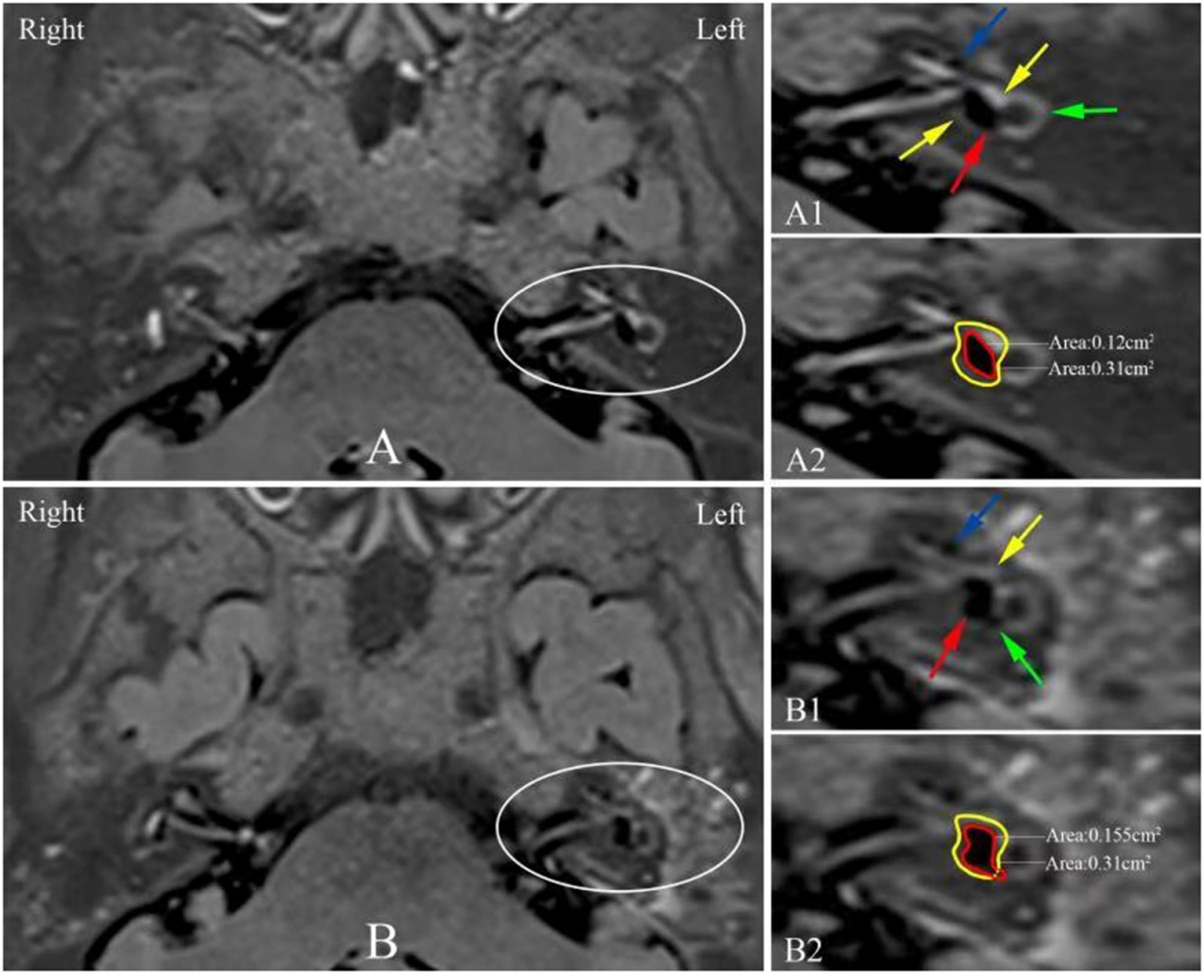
3D-real IR MRI showing a dynamic change of EH in the left ear of the patient no. 8 (Table 1) between before (A) and after surgery (B). (A1) The image shows black EH (red arrow) is surrounded by white perilymph in the vestibule (yellow arrow) and a mild hydrops can be detected in the basal turn of the cochlea (blue arrow) where the lateral semicircular canal ring was visualized >240° (green arrow). (A2) The red solid trace indicates the endolymphatic space and the yellow solid trace indicates the fluid space in the vestibule, where the vestibular hydrops ratio (VHR) is 39%. (B1) Postoperative image shows the vestibular hydrops (red arrow) is increased and white perilymph space is reduced (yellow arrow), where the increased EH herniation into the non-ampullated side of LSCC (green arrow) and a significant hydrops in the basal turn of the cochlea (blue arrow) are observed. (B2) The red solid trace indicates the endolymphatic space and the yellow solid trace indicates the fluid space in the vestibule, where the VHR is 50%. EH, endolymphatic hydrops; LSCC, lateral semicircular canal; 3D-real IR, three-dimensional real inversion recovery; MRI, magnetic resonance imaging; MD, Meniere's disease.
